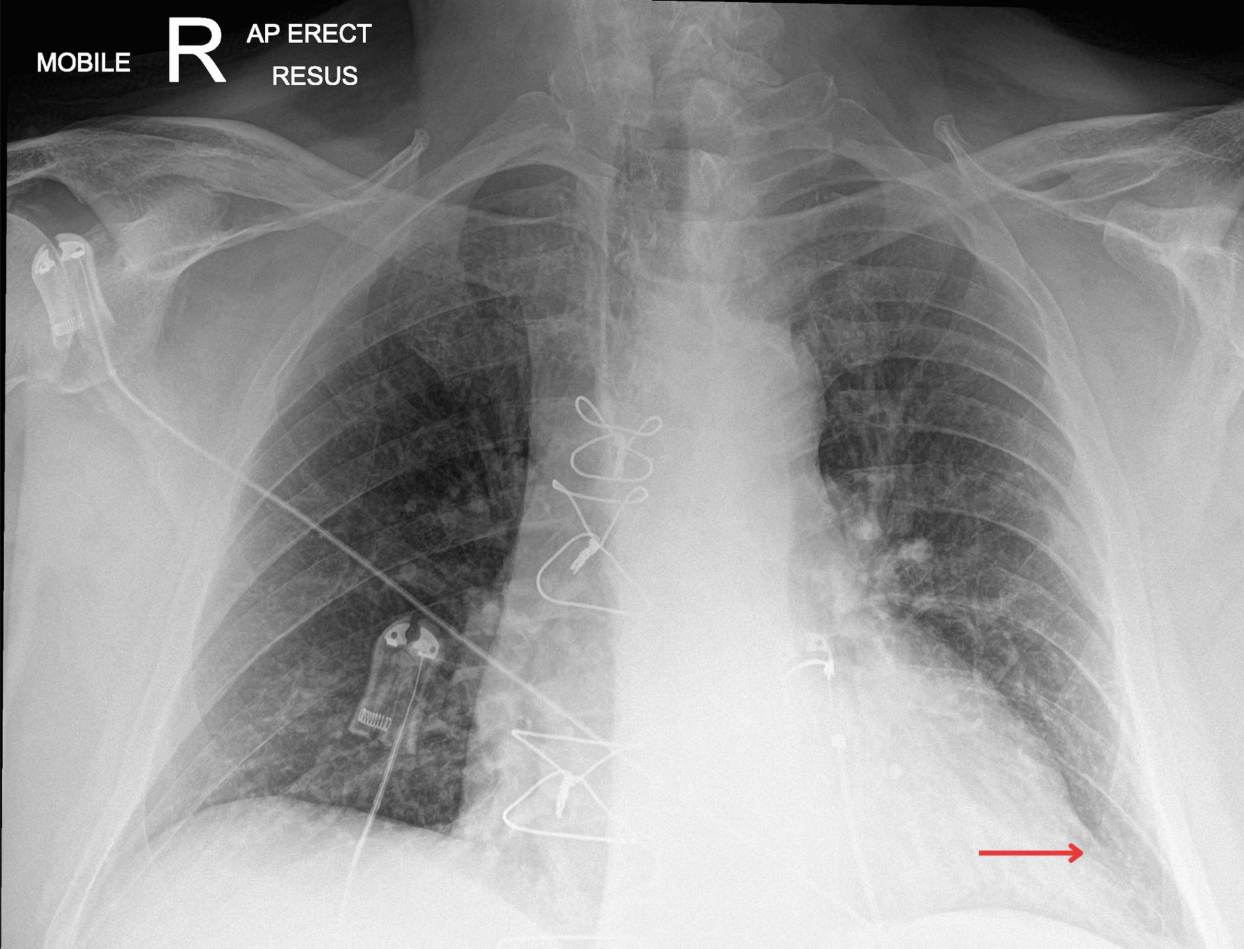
Admission chest X-ray. The arrow showing the area of suspected consolidation.
